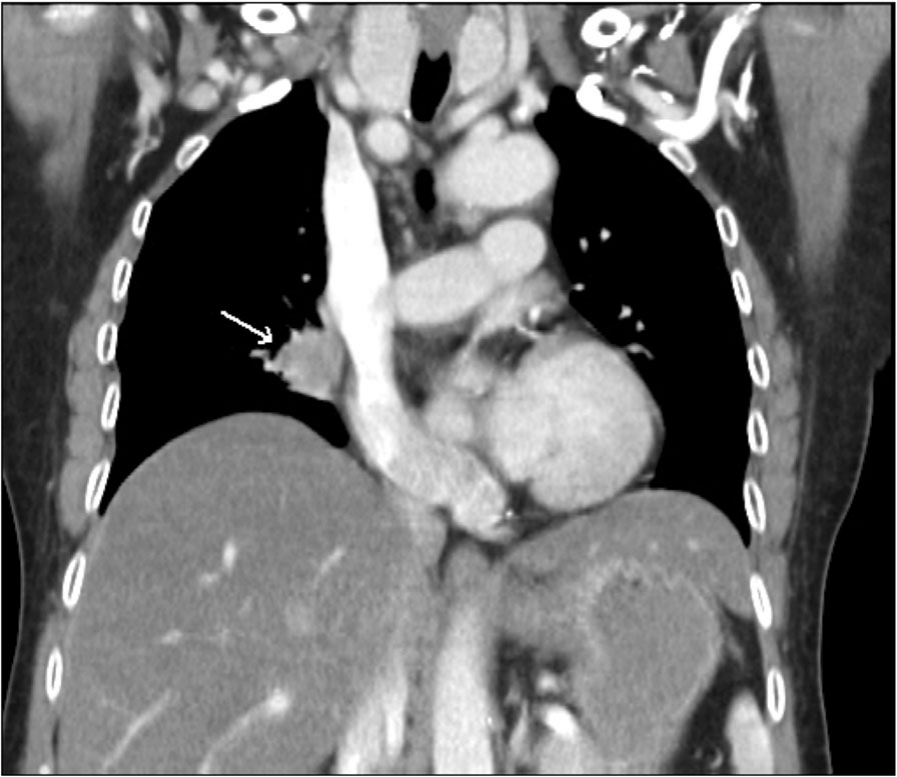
CT chest with contrast revealing an enhancing soft tissue mass occupying the proximal part of bronchus supplying the medial segment of right middle lung (arrow)
